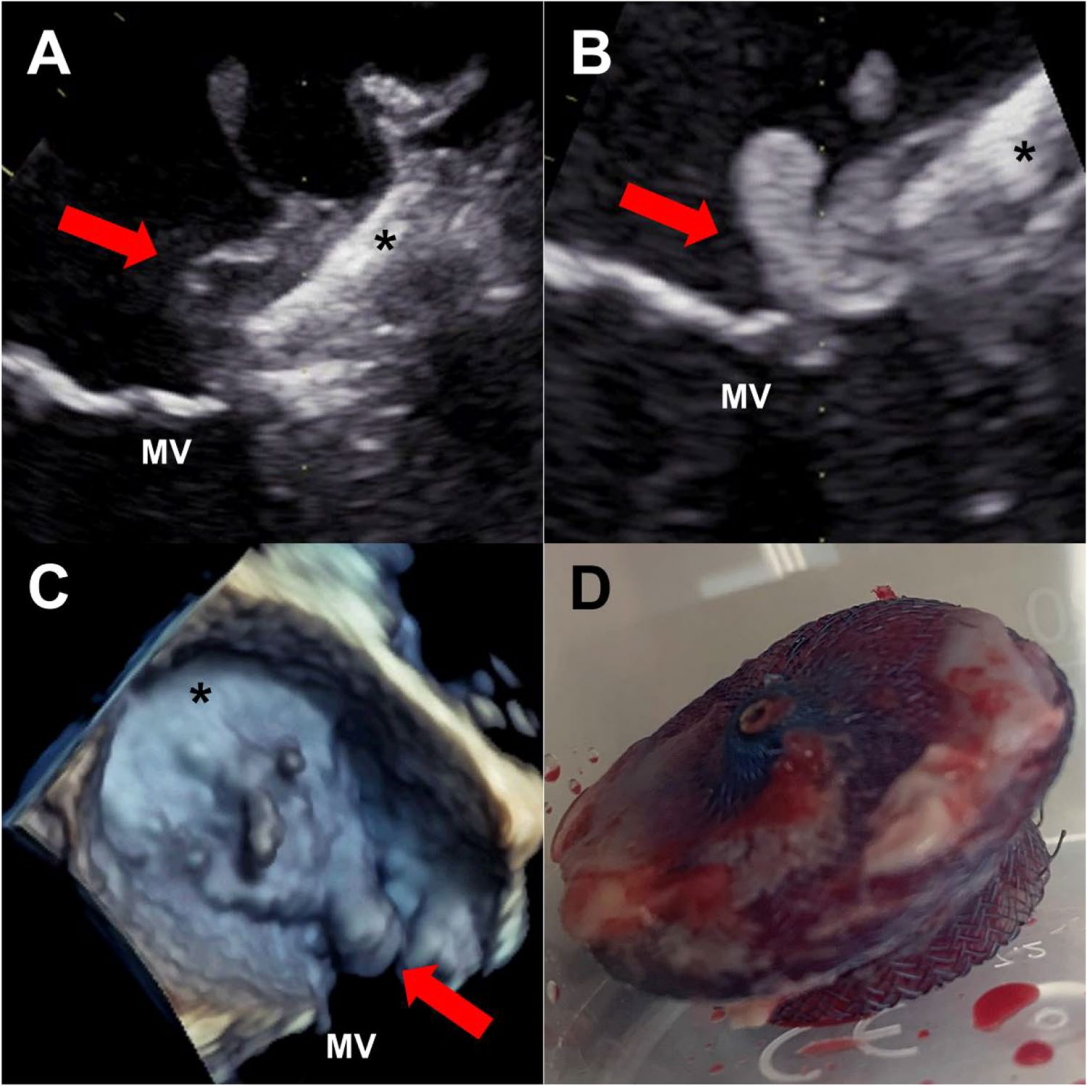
a Transesophageal echo (TEE) post transvenous lead extraction revealing a gelatinous mass (red arrow) on the LAAO (asterisk) in close proximity to the mitral valve (MV). b Control TEE showing persistence of the mass with growth (red arrow) towards the mitral valve (MV). c 3-D-image of the LAAO (asterisk) with the growing mass on the device and in the anterolateral commissure (red arrow) of the MV. d Retrieved device showing endothelial growth and purulent infection
